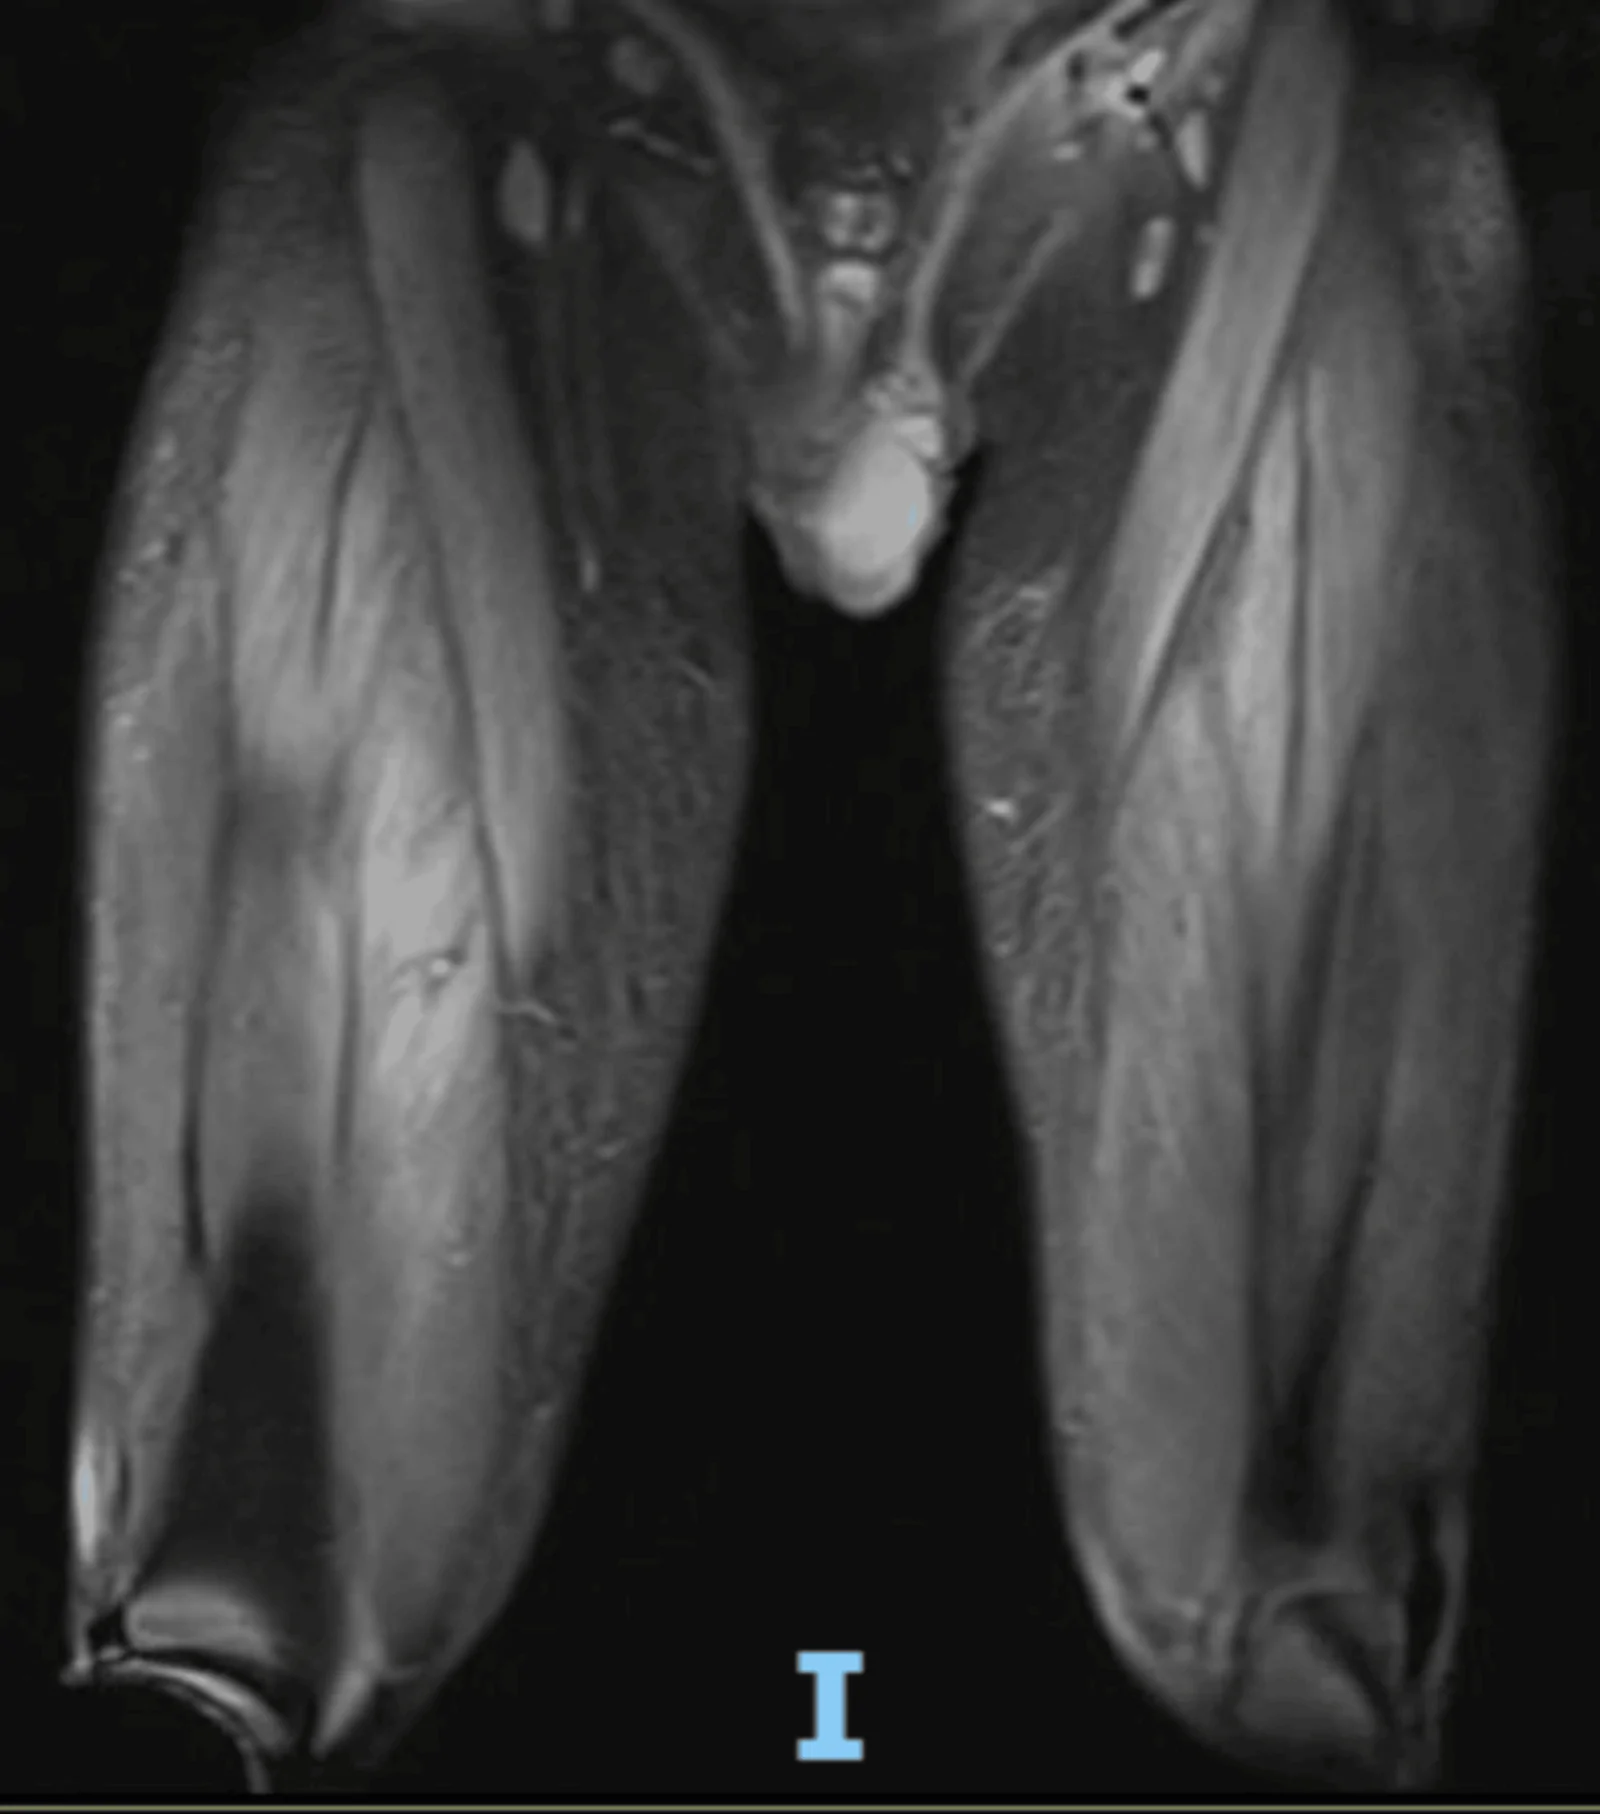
MRI lower-extremity non-joint with/without IV contrast MRI lower-extremity non-joint with/without IV contrast showing diffuse high short TI inversion recovery (STIR) signal with associated enhancement involving bilateral thigh musculature, most prominent in the mid to distal portion of the right sartorius muscle, right mid semimembranosus muscle, and left rectus femoris.
